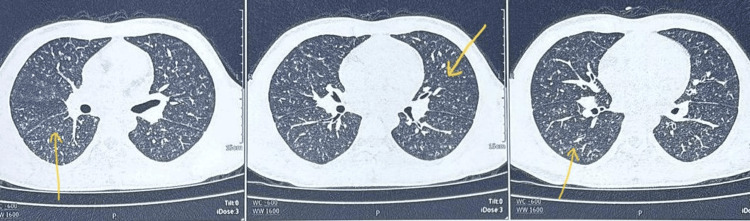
HRCT thorax in Case 2 showing diffuse centrilobular nodules HRCT: High-Resolution Computed Tomography The yellow arrows are showing bilateral diffuse centrilobular nodules.
